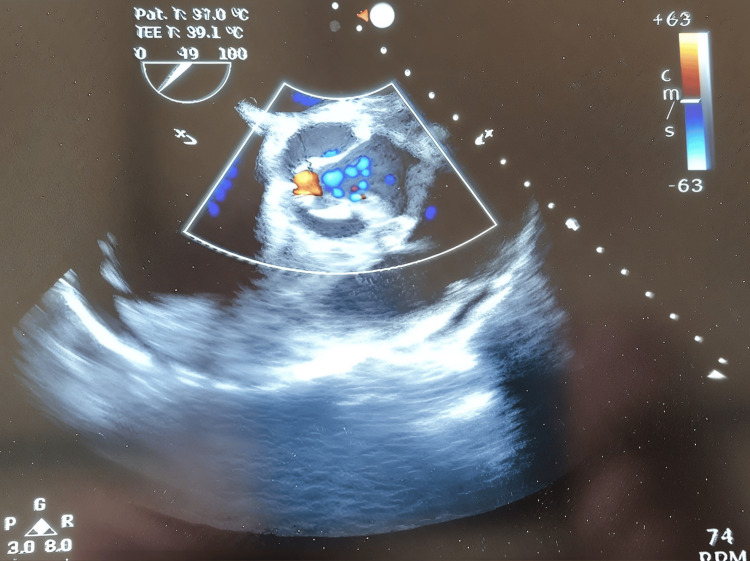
Aortic root abscess (as identified by perioperative transesophageal echocardiography).
